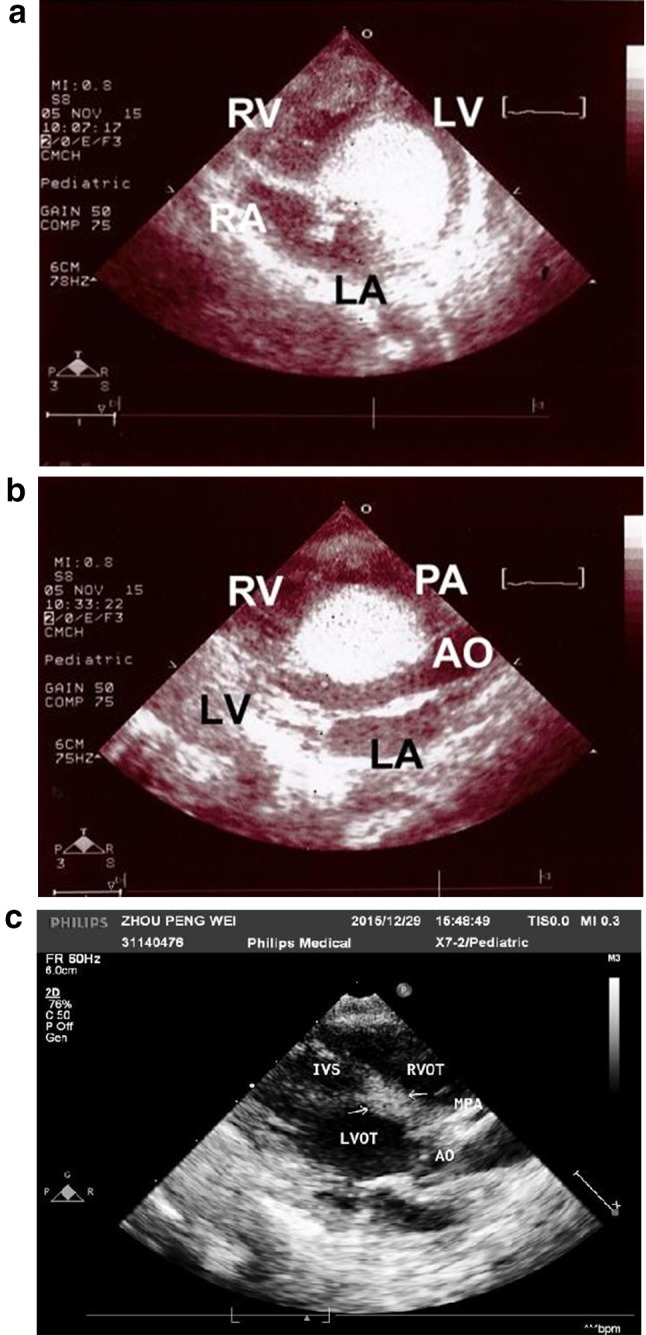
Case 3: a, b Echocardiogram on first day of life. c Echocardiogram after 2 months of everolimus therapy
